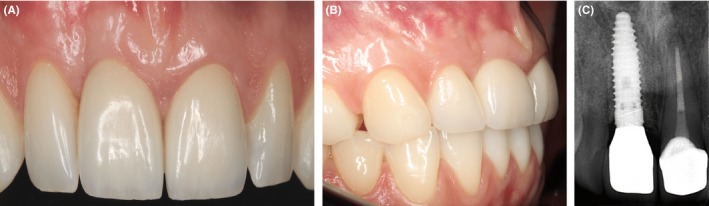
Postoperative assessment of the implant placement after one year. A and B, one‐year post‐op. C, shows the radiographic assessment one‐year post‐op. No change in position of attached gingiva and zenith
